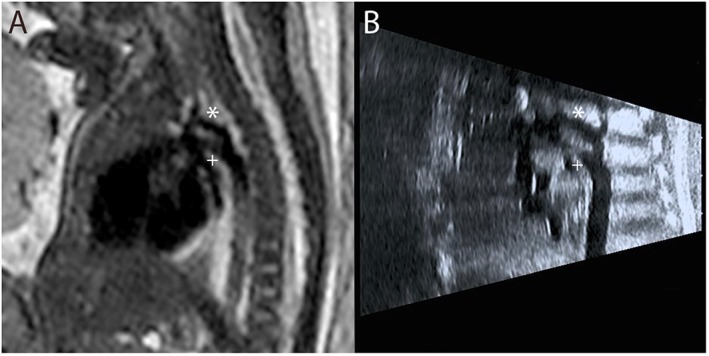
Single‐shot fast spin‐echo (SSFSE) black‐blood image in a 32‐week fetus with suspected coarctation of the aorta. The hypoplastic aortic arch (*) is visualised superior to the dominant ductal arch (+) on fetal MRI (A), confirming prenatal ultrasound findings (B)
